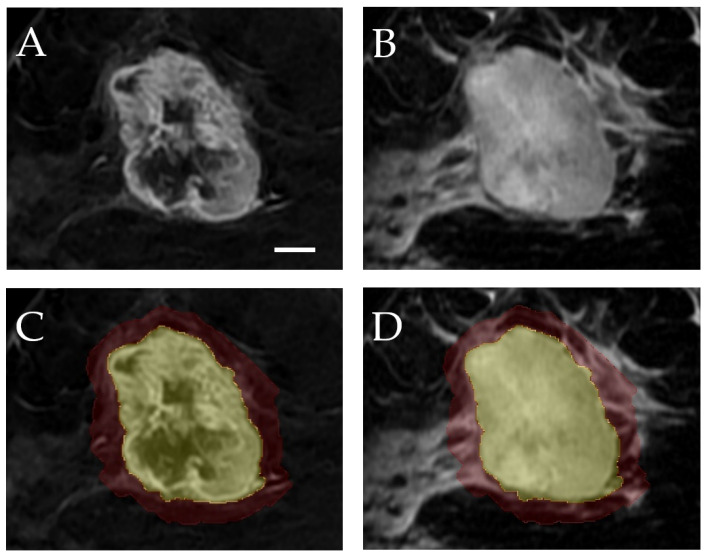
Visualization of tumor segmentation in MR images. (A) Contrast-enhanced T1-weighted (CE-T1) image; (B) T2-weighted image; (C) CE-T1 image with intratumoral (yellow) and peritumoral (red) segmentations; (D) T2 image with the same segmentations. A scale bar corresponding to 1 cm is shown in the bottom right corner of image (A).
